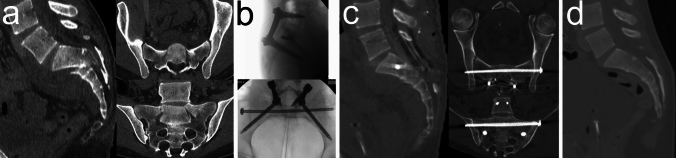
Spinopelvic dissociation injury (SPDI; Type 2 TLSI). A 19-year-old patient sustained an SPDI with cauda equina dysfunction (Gibbons Grade 4) following a suicidal jump. a Preoperative CT images showing a U-shaped Type 2b SPDI with the transverse fracture component at S2. b Intraoperative fluoroscopic images after open decompression (including sacral laminectomy), reduction, and TLPF (with S2 alar-iliac screws). c Postoperative multiplanar CT reconstructions demonstrating realignment of the central upper sacrum compared with d a preinjury CT scan available for this patient
